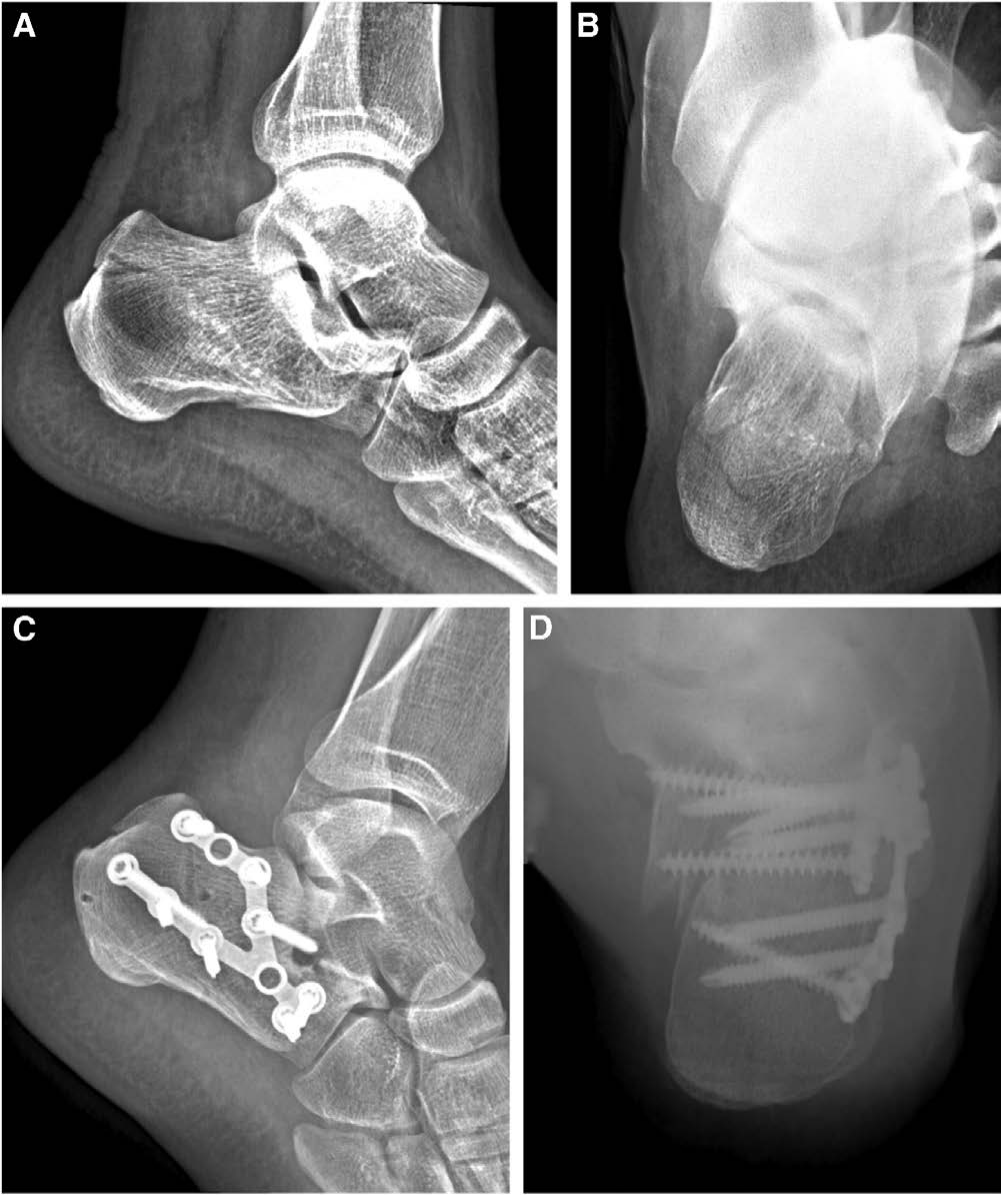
A 37-year-old male patient fell from a height of 1.5 m. (A and B) Preoperative radiograph showing left calcaneal fracture (Sanders type IIB) with unnormal Böhler angle (27°), Gissane angle (112°), height (81.4 mm), length (83.3 mm), and width (54.8 mm). 2-arm straight plate fixation via the sinus tarsi approach was used. (C) Postoperative lateral radiograph shoes well restoration of the Böhler angle (25°), Gissane angle (113°), height (86.7 mm), and length (82.3 mm). (D) However, the axial radiograph shows a broadening body (52.6 mm), accompanied by misalignment and varus deformity. AOFAS score was 82 at the last follow-up. AOFAS = American Orthopedic Foot and Ankle Society.
